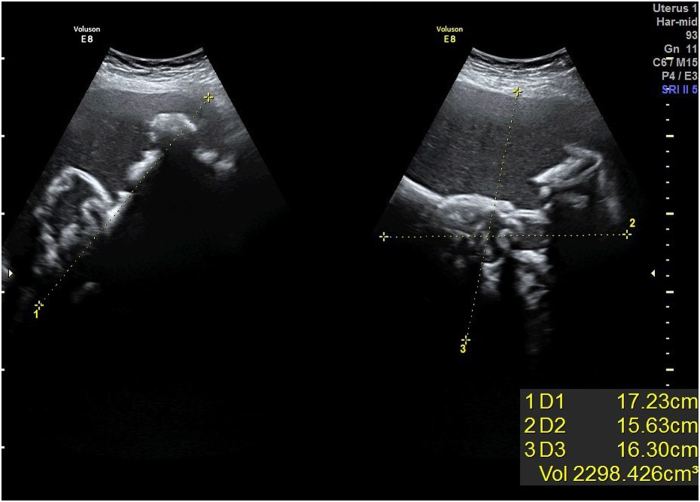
Transabdominal sonography of 56-year-old female reveals large cystic lesion with internal echo and linear echogenic regions with posterior acoustic shadow in dependent part.
